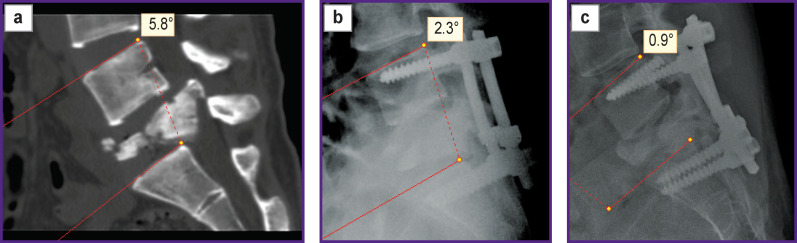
CT scan and X-ray examination of patient R., 35 years old: (a) 10 days after injury; (b) 6 months after transpedicular fixation; (c) 12 months after transpedicular fixation. Attention should be paid to a decrease in the L_4_–S_1_ regional angle in the postoperative period up to 6 months. Instability of the metal structure developed 12 months after surgery
